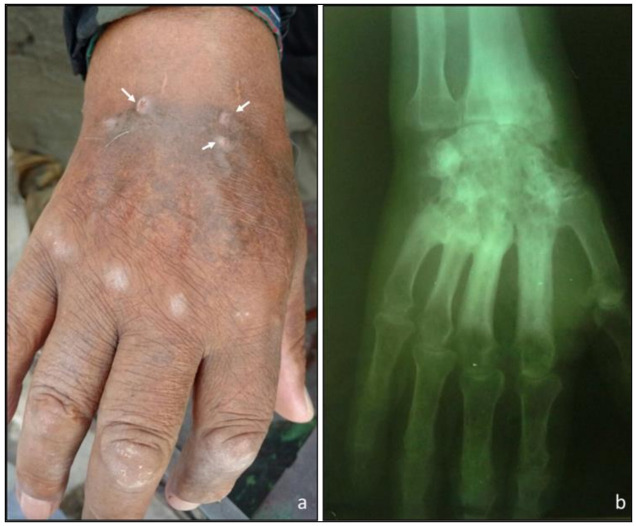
Subcutaneous infection of the right hand: arrows indicate drain sinuses produced in the lesion (a). X-ray of hand showing bone lesions (b).
